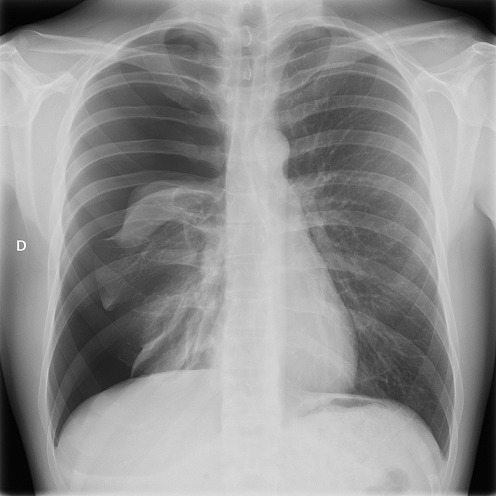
Thorax X-ray at the arrival
